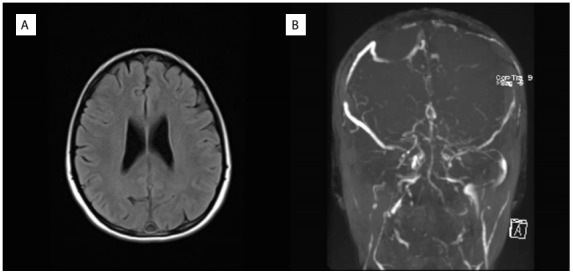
a) Cranial magnetic resonance imaging; b) Magnetic resonance imaging venography.
